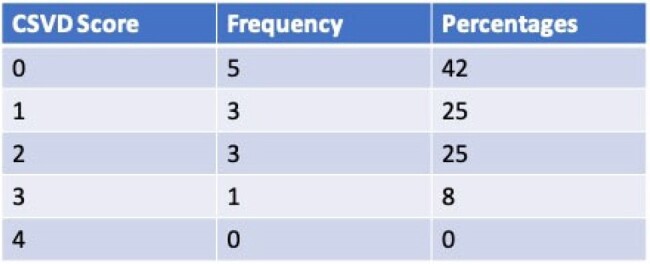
Cerebral Small Vessel disease Score in PWH with substance use disorder. 5 participants had a CSVD score of 0 but "age related white matter disease" on Brain MRI
